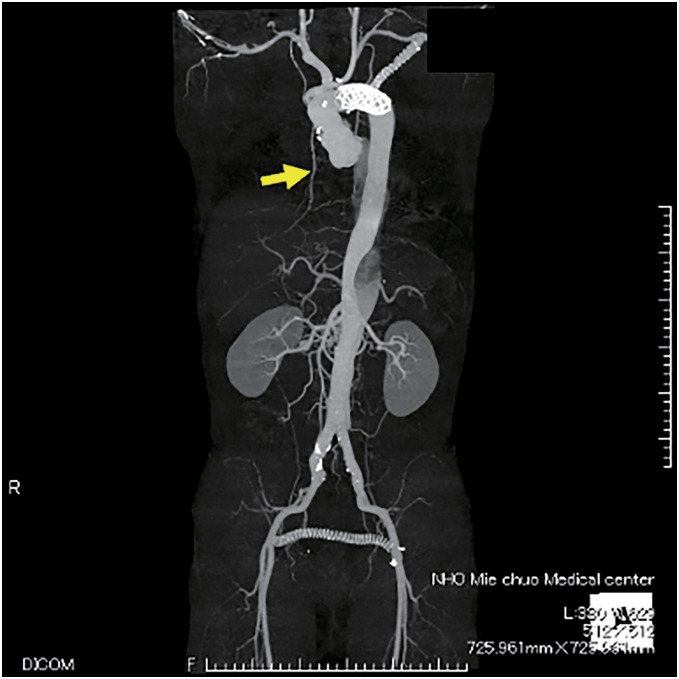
Fig. 1 Preoperative CT angiography. Yellow arrow shows the right IMA enhanced. CT: computed tomography; IMA: internal mammary artery
